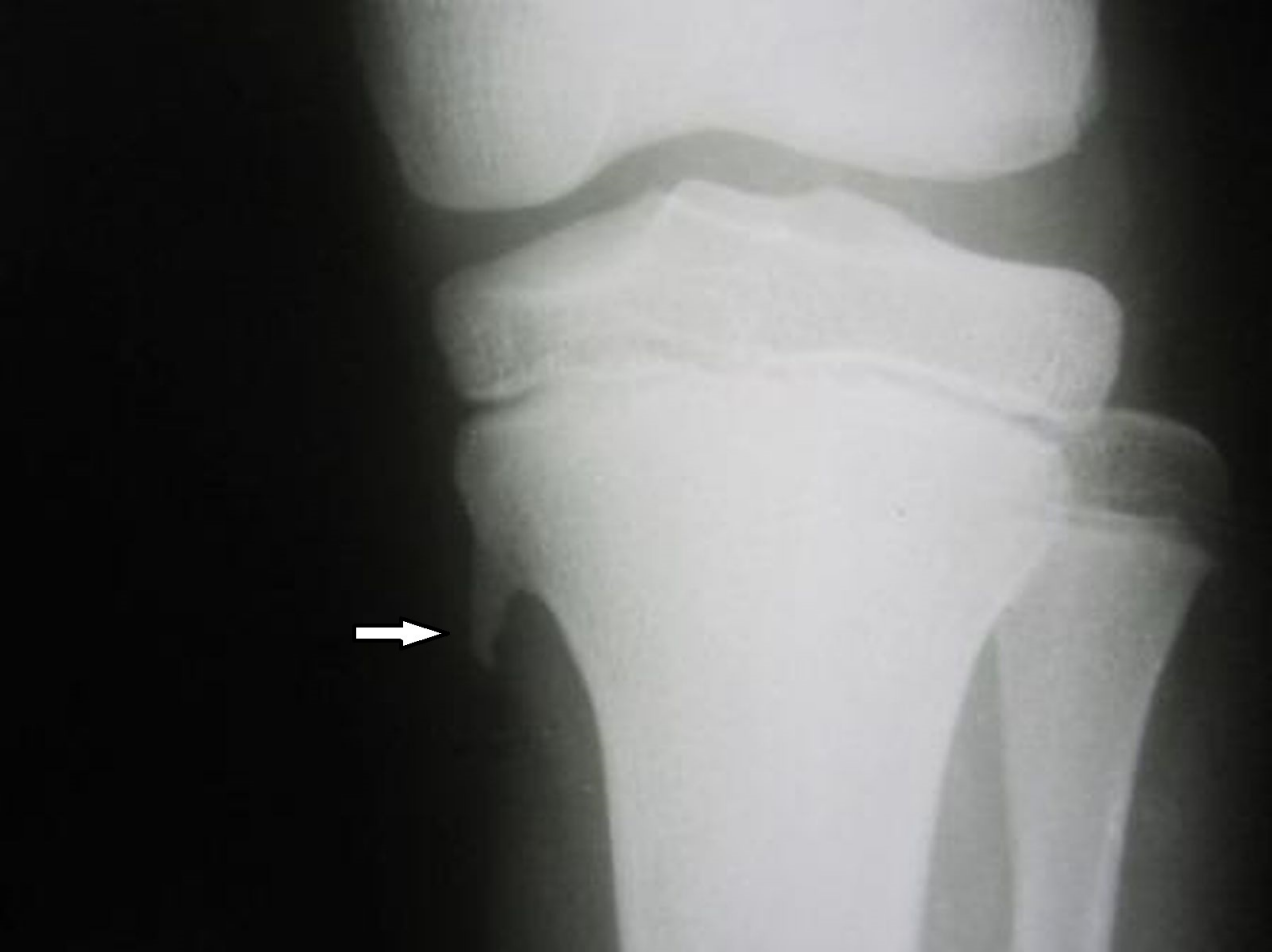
Case 2: Plain radiograph of the left knee—anteroposterior view Sharp bony spur (white arrow) arising from proximal tibial metaphysis, similar to the first case.
